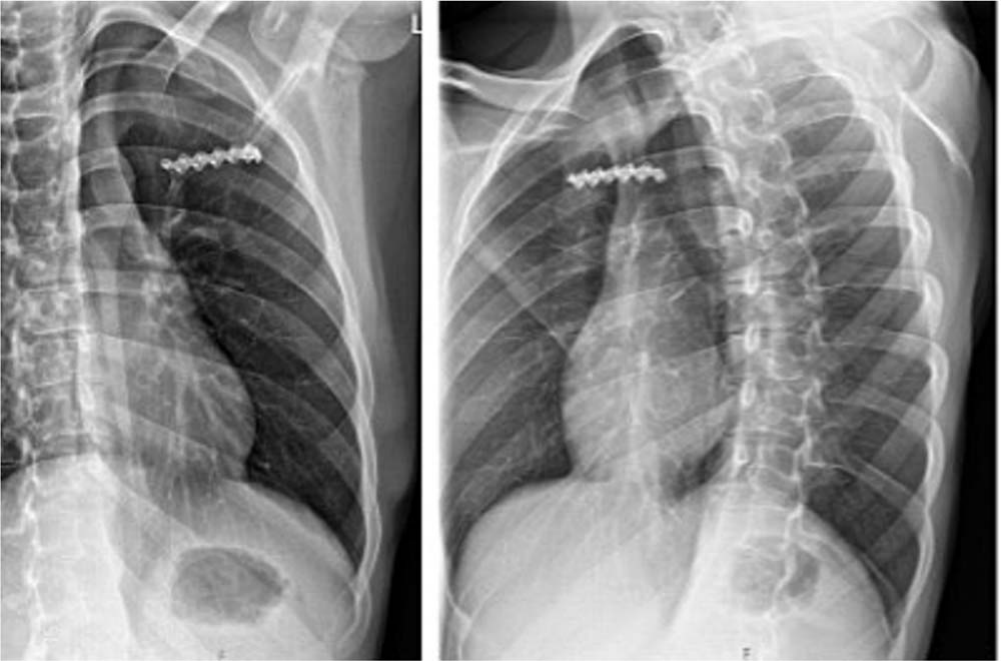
Postoperative X-ray showing the bridging position of the plate on the third rib.
